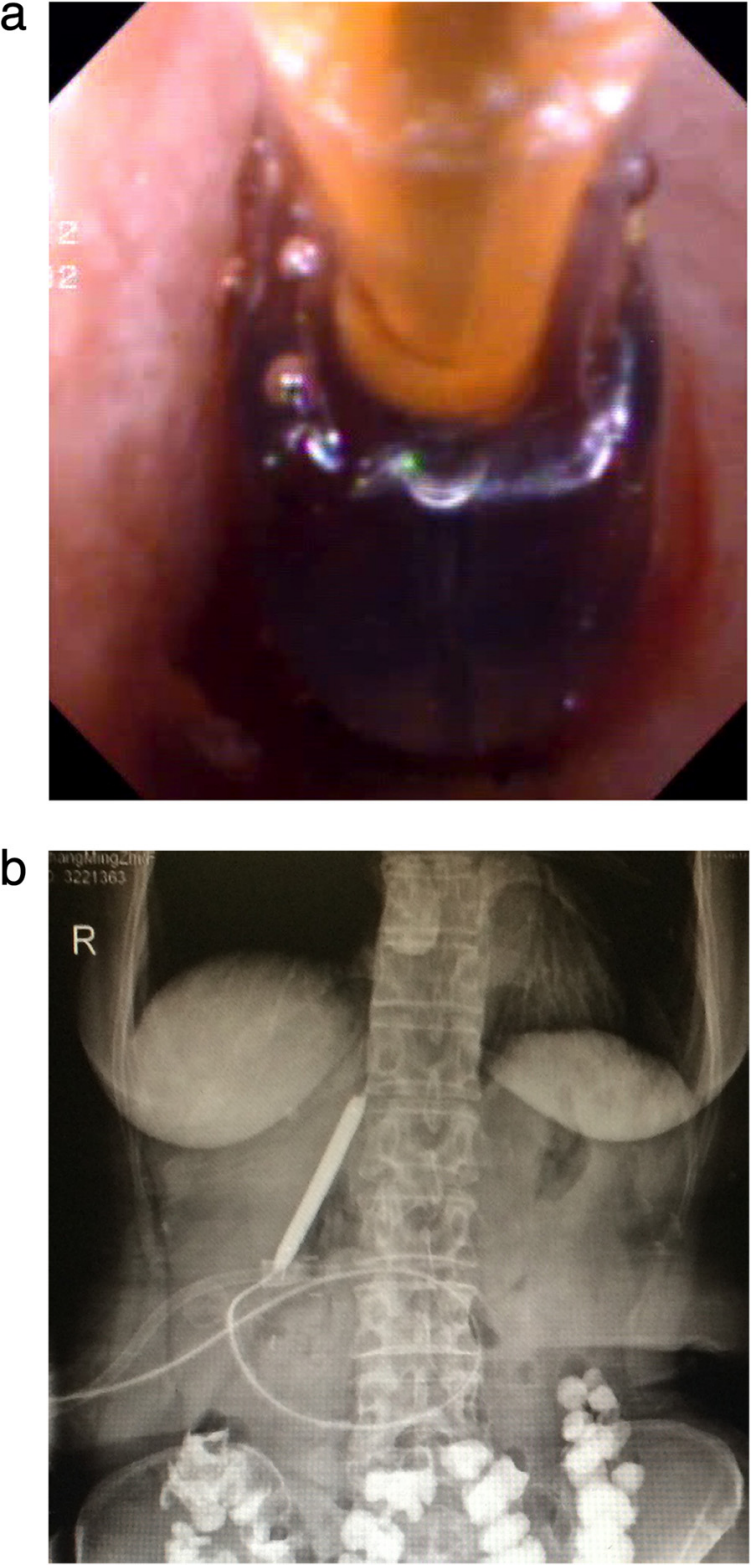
Balloon dilation of anastomotic strictures: a) Choledochoscopic balloon dilatation of the anastomotic stricture under the guidance of the yellow zebra guide wire. b) Balloon dilation under X-ray
